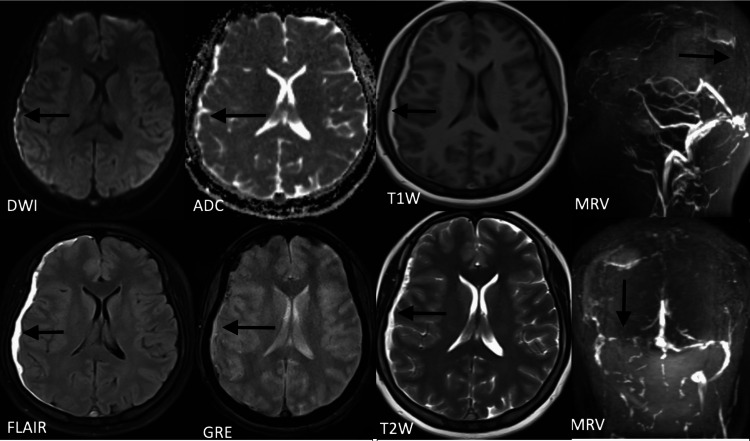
Magnetic resonance imaging of the brain (T2/FL) Air sequence showing crescent-shaped extra-axial hyperintensity and loss of flow void in superior sagittal sinus and few cortical vein thrombosis. Magnetic resonance imaging of the brain venogram suggests superior sagittal sinus, right transverse, and few cortical vein thrombosis. DWI: Diffusion-weighted imaging, ADC: Apparent diffusion coefficient, FLAIR: Fluid-attenuated inversion recovery, GRE: Gradient recalled echo, MRV: Magnetic resonance venogram.
